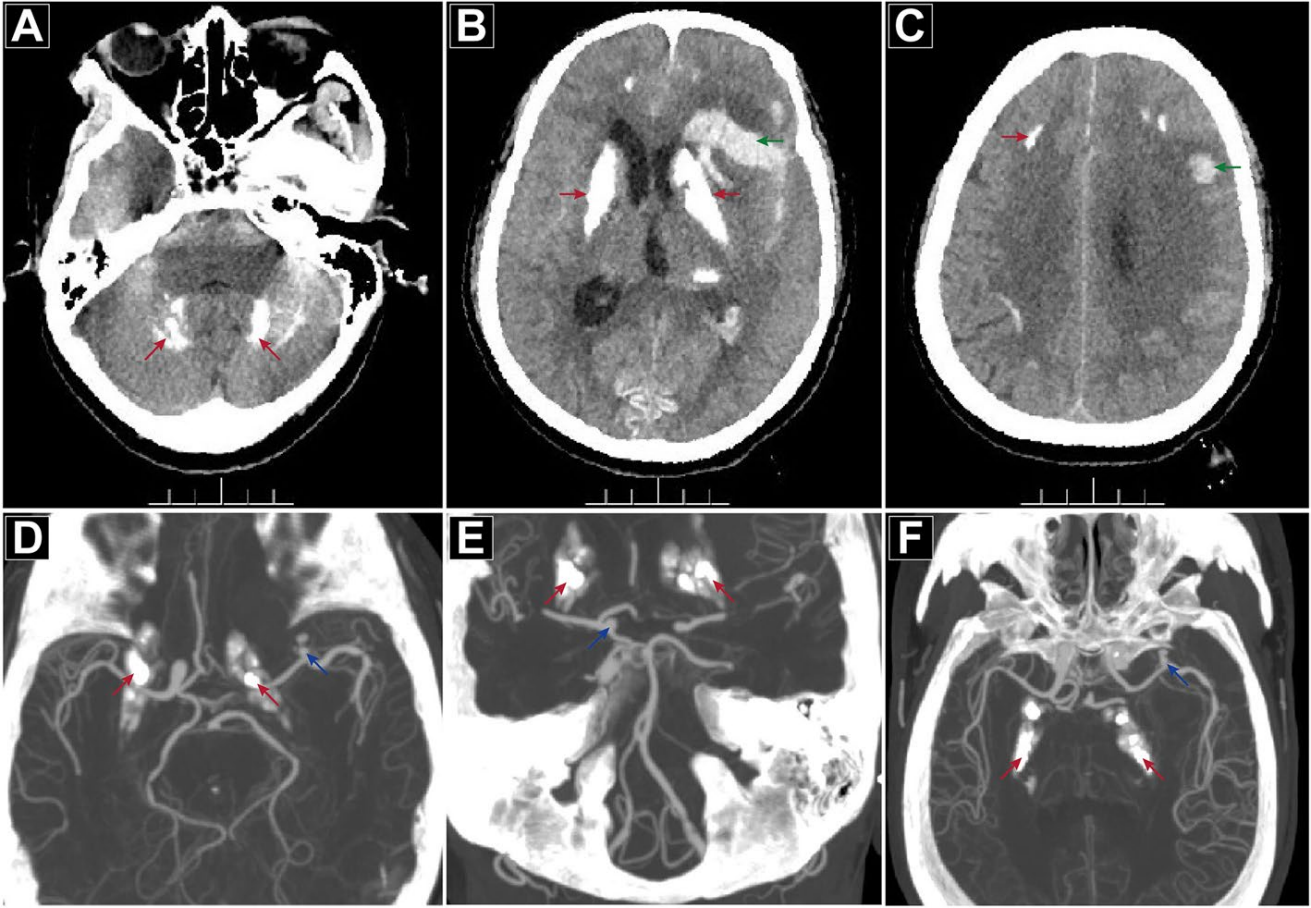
CT scan and CTA at admission
